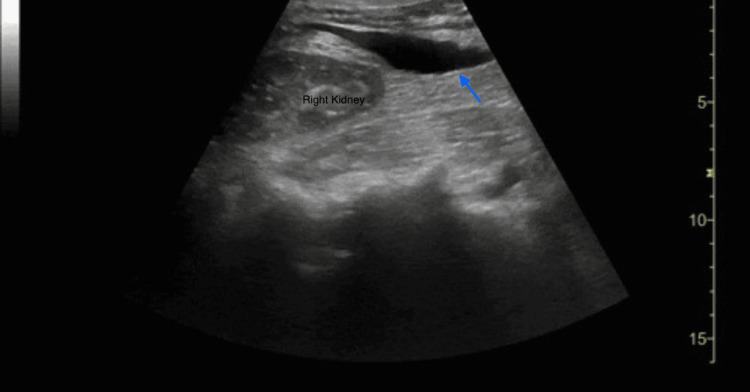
RUQ abdominal ultrasound near the caudal liver tip with free fluid. Anechoic fluid identified by the blue arrow just past the caudal tip of the liver and adjacent to the right kidney. RUQ, right upper quadrant
